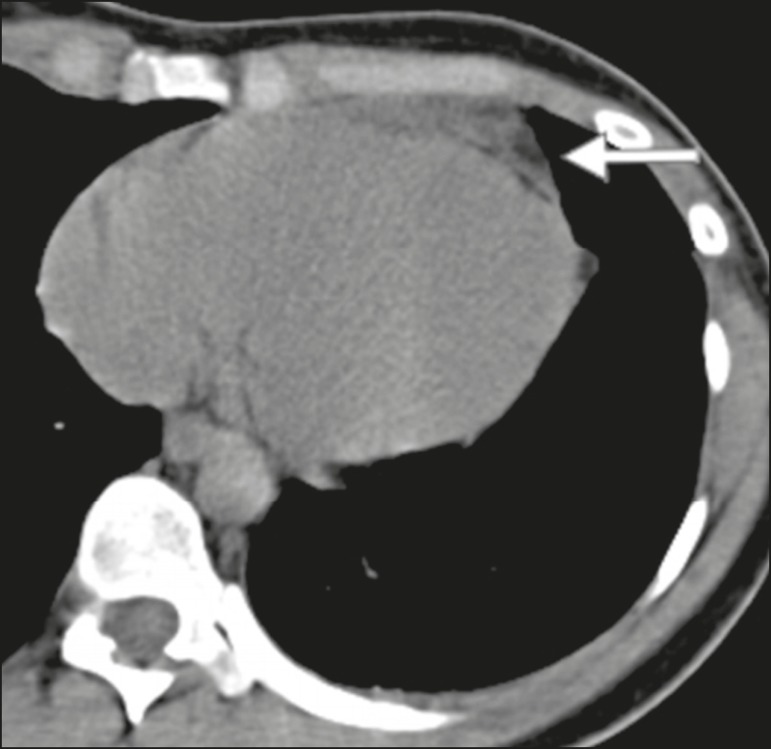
CT scan of a 29-year-old female with acute pleuritic chest pain showing a
